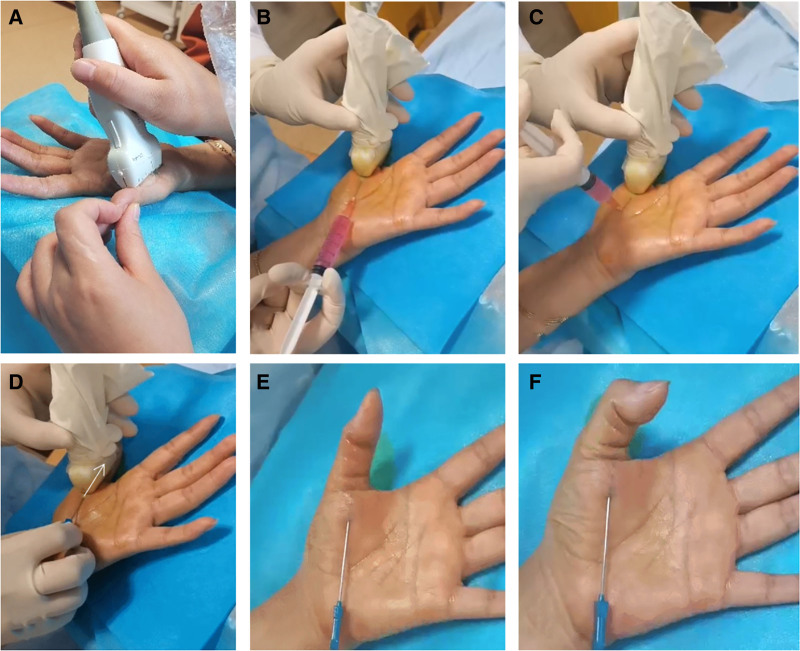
(A) Location of ultrasound examination; (B, C) local injection of infiltration anesthesia; (D) ultrasound-guided acupotomy longitudinal cutting; (E, F) flexion and extension of the left thumb after acupotomy treatment.
